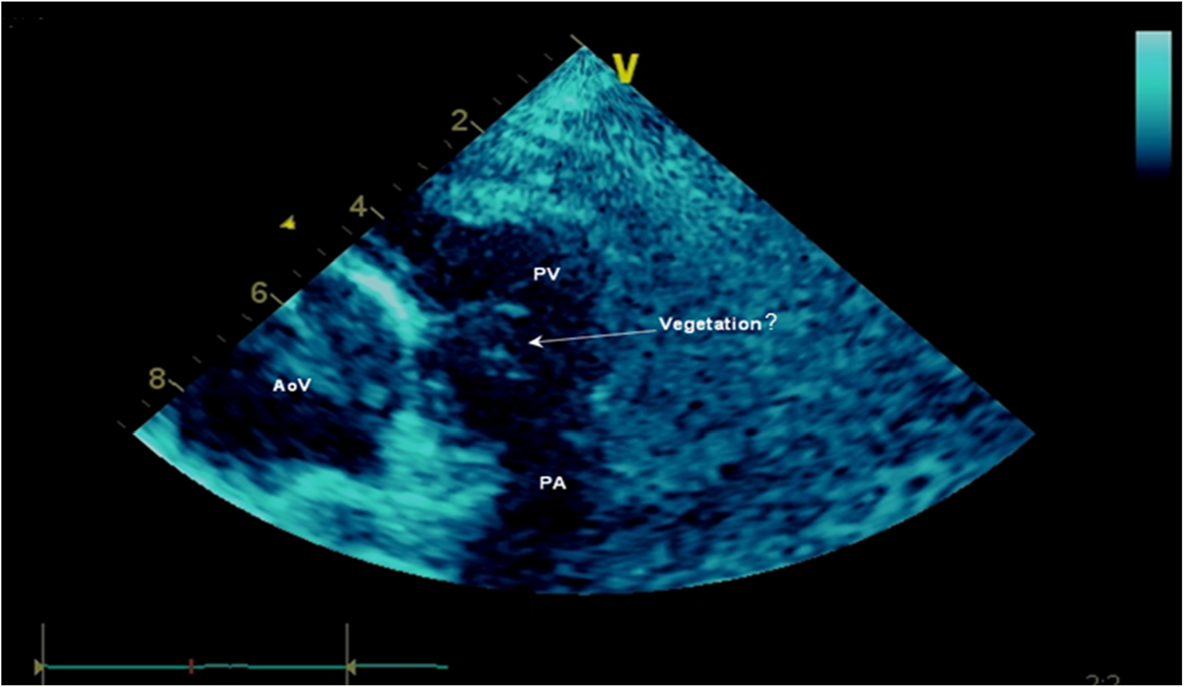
Transthoracic echocardiogram revealing an erratic structure in the pulmonary valve suggestive of vegetation. AoV, aortic valve; PA, pulmonary artery; PV, pulmonary valve.
